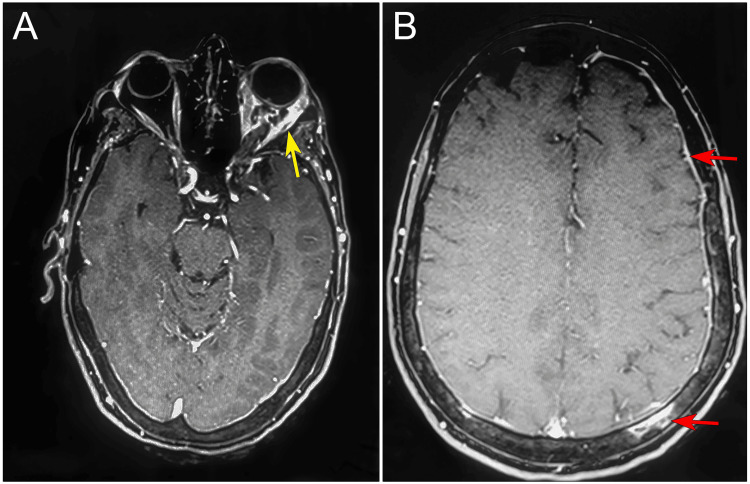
MRI imaging. (A) Axial contrast-enhanced MRI with the window set at the orbital level; the yellow arrow shows fat-suppressed orbital infiltration on the left with extensive contrast impregnation involving the extraocular musculature. (B) Axial contrast-enhanced MRI with the window set at the cranial level; the red arrows show fat suppression demonstrating metastasis in the left parietal bone and increased thickness of the dura mater, suggesting meningeal carcinomatosis.
